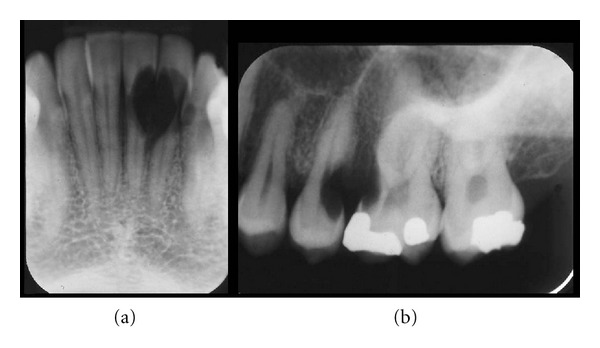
Patient 2: radiographs demonstrating regional aggressive root resorption in the left mandibular incisors (a) and in the left maxillary second premolar and first molar (b).
